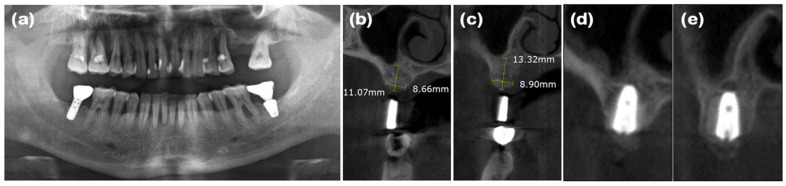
Radiographs of case 2. (a) The pre-operative panoramic view taken during the initial visit. (b,c) CBCT images displaying the augmented site after healing. The alveolar ridge width was measured at approximately 8.66 and 8.90 mm on the maxillary right first and second molar areas, respectively, which is sufficient for the placement of a standard diameter implant. (d,e) Implants were placed in the maxillary right first and second molar areas, respectively.
